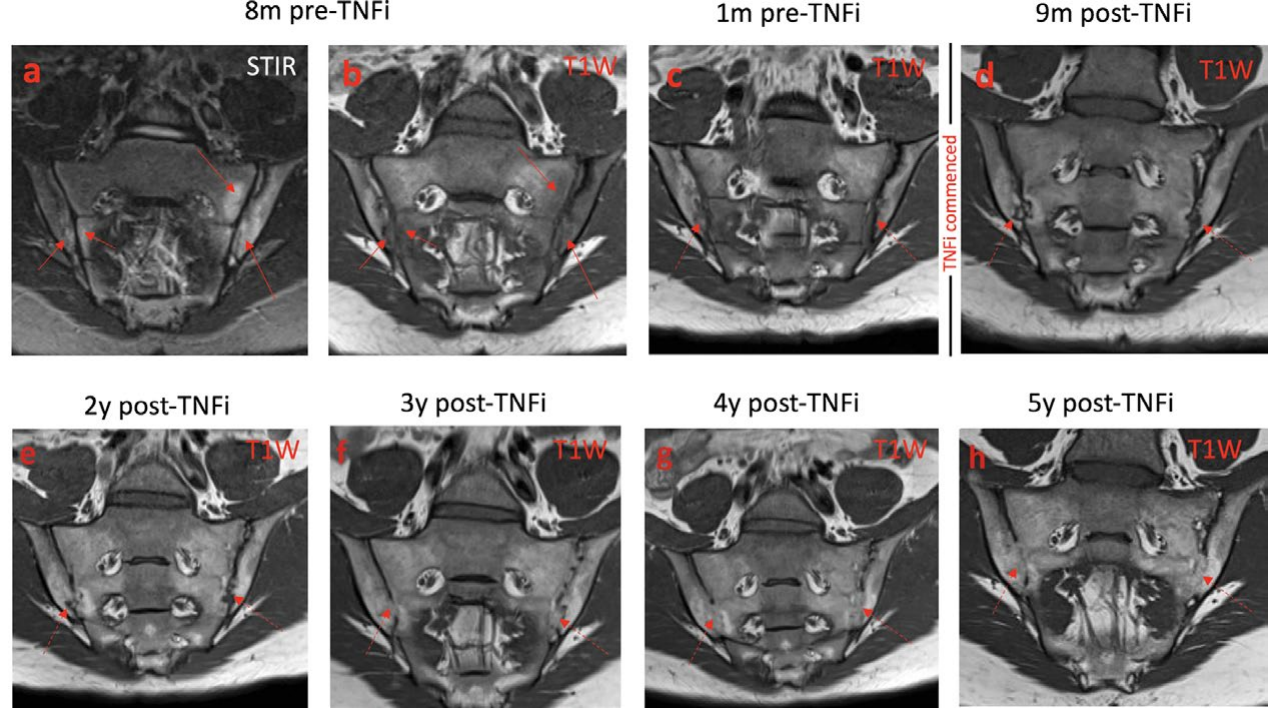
Magnetic resonance images from a representative patient showing the progression of ankylosis over a 5‐year period. a–c, Before the initiation of tumor necrosis factor inhibitor (TNFi) therapy, bilateral bone marrow edema is evident on the STIR image (a) and T1‐weighted (T1W) images (b and c). d–h, After TNFi therapy, the joint erosions gradually become less distinct, and the joints ultimately fuse in anatomic locations similar to those in which the initial edema was found. Arrows indicate the areas of edema (on STIR images) and fat metaplasia (on T1W images).
